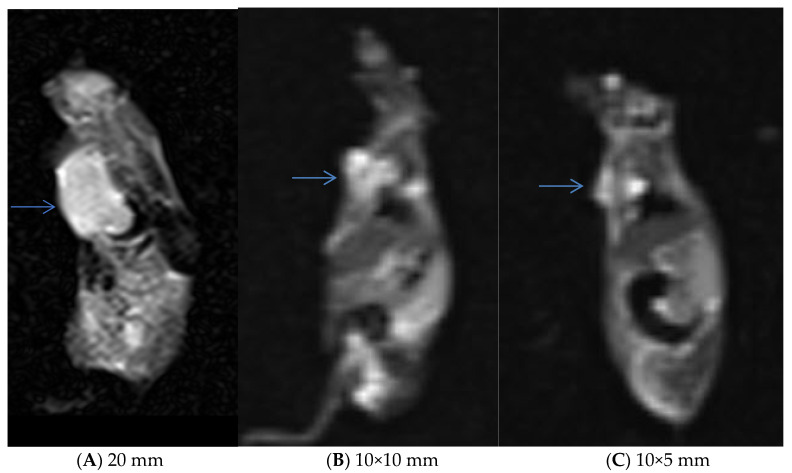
The second MRI performed 6 days after the initiation of the treatment. Stable size of the mass in the control, blue arrows show tumors (A) and a significant decrease in the size of the lesions in the treated tumors (B,C) (“(B)” was the case treated with MTX, and “(C)” was treated with MNP@MSN-MTX in the presence of external magnet).
